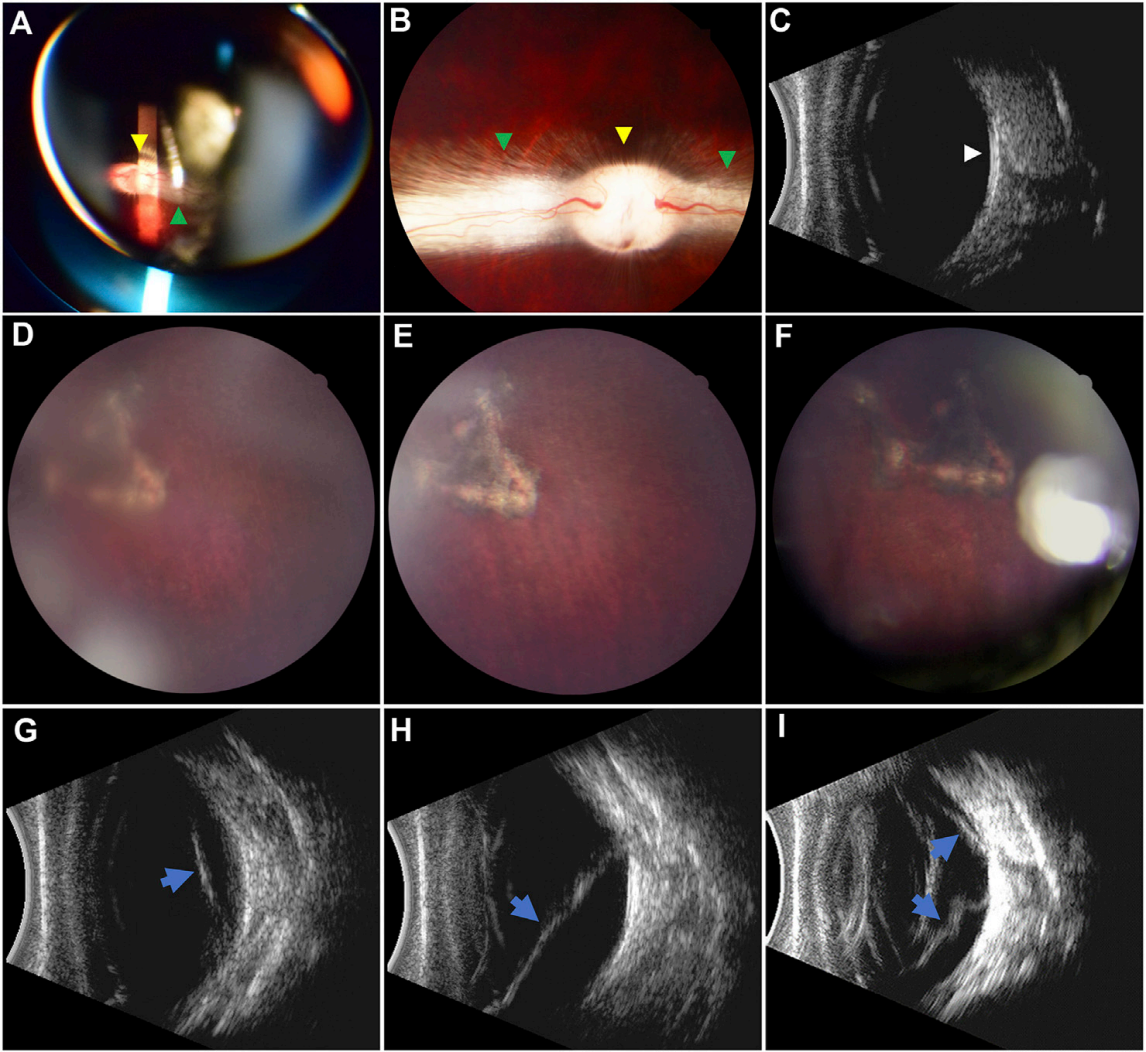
Pre- and postoperative fundus examination. (A–C) Preoperative fundus examination *via* indirect ophthalmoscopy, fundus photography, and B-mode ultrasound, respectively. The optic disc (yellow arrowheads) and myelinated nerve fibers (green arrowheads) were well-marked. The vitreous cavity was clear, and the retina was attached (white arrowhead) in the ultrasound image. (D–F) Retinal reattachment in the RRD-hydrogel group at 7 days and 1 and 3 months after surgery, respectively. Pigmentation around the retinal break was apparent. (G–I) Retinal detachment (blue arrows) in the RRD group at 7 days and 1 and 3 months after surgery, respectively. The localized retinal detachment (G) extended (H) and gradually became stiff from a soft state over time (I).
